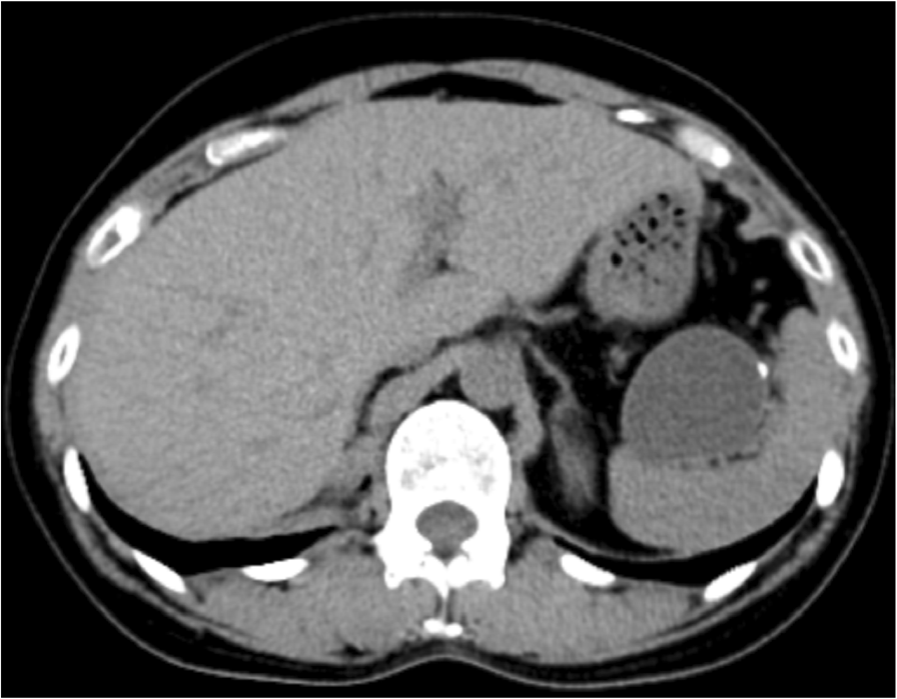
A thick cystic lesion of the septum is visible in the pancreatic tail, but computed tomographic scan shows no invasion into the stomach wall
